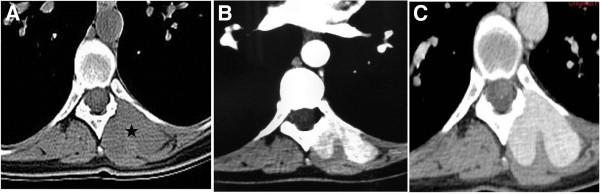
Unenhanced CT (A) demonstrates a deep soft tissue mass in the left paravertebral area (asterisk). The mass is isodense compared to muscle. No bone erosion is observed. At 30 s post-contrast infusion (B) the lesion exhibits mild heterogeneous enhancement while at 90 s post-contrast infusion (C) the lesion shows more intense homogeneous and prolonged enhancement.
